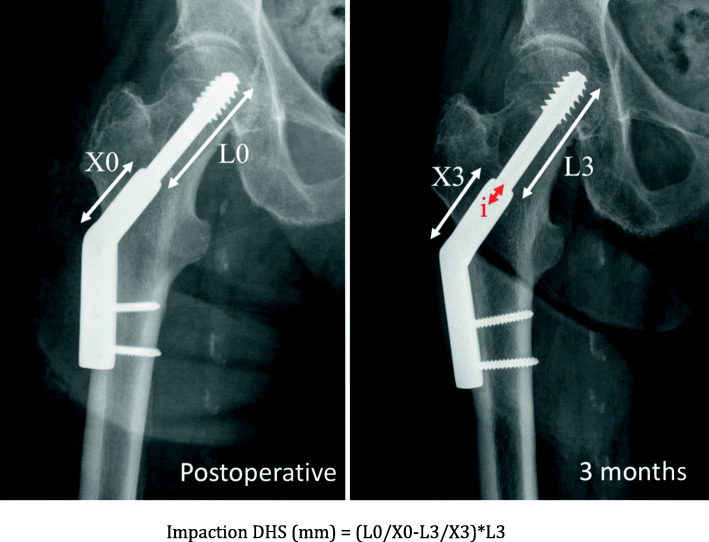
Calculation of impaction with DHS implant. Postoperative and 3-month X-ray
